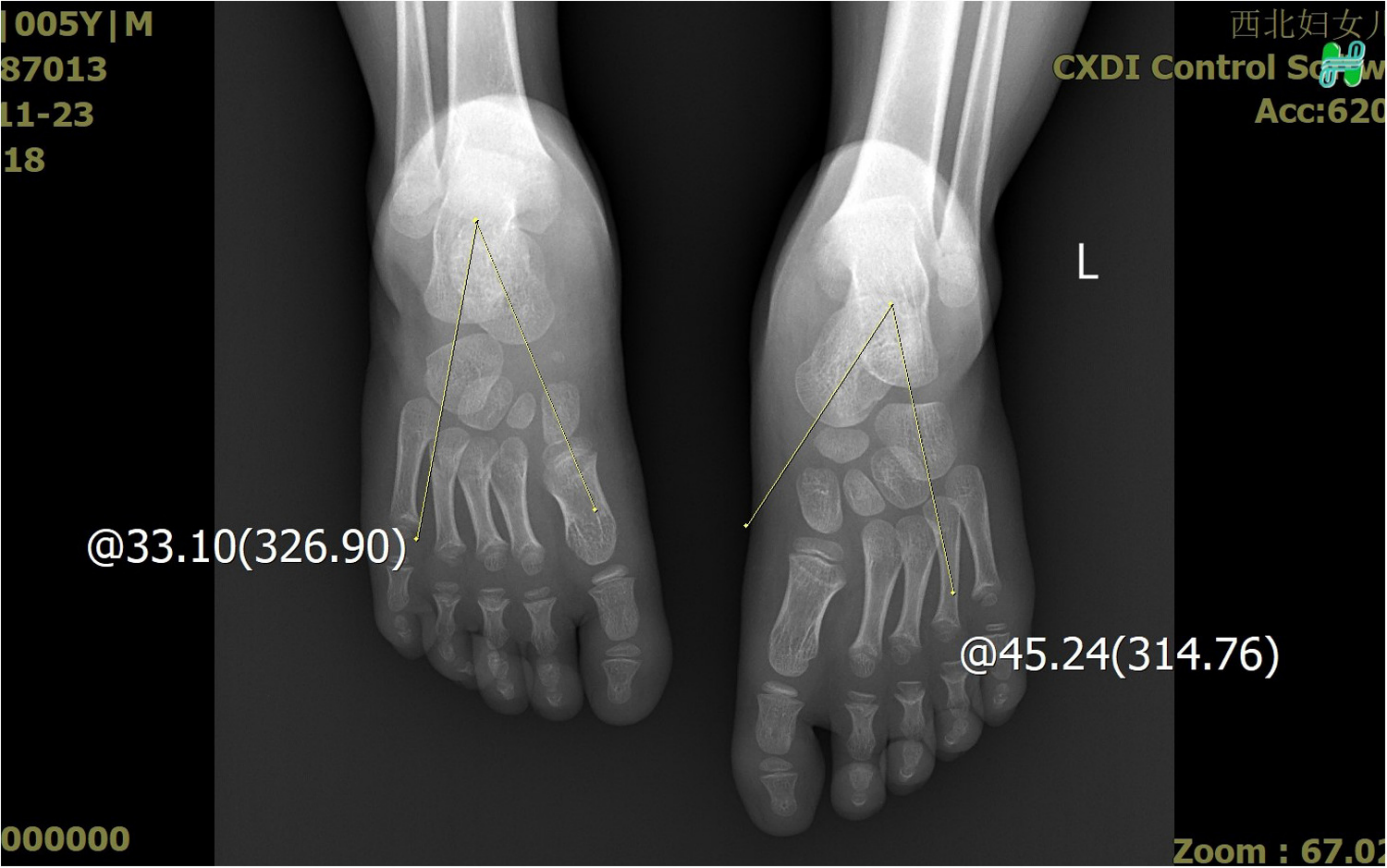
The X-ray demonstrated that the affected talocalcaneal angle was smaller than the normal side.
